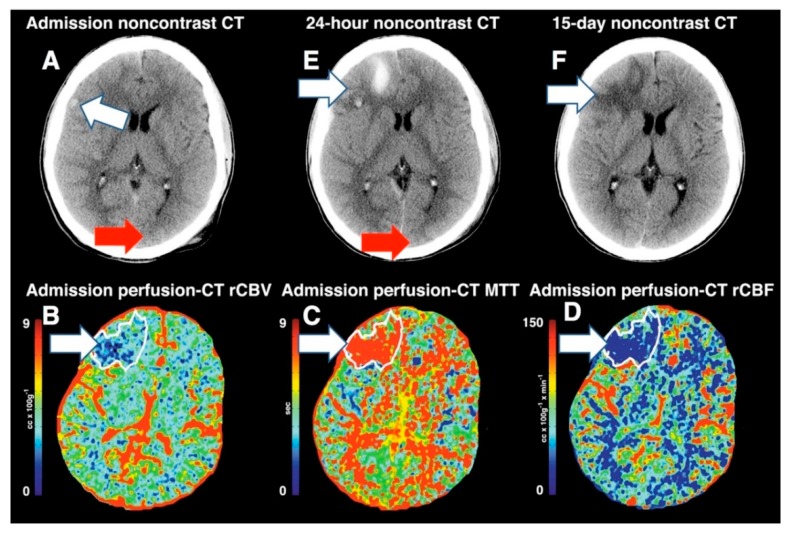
Noncontrast and perfusion computed tomography (PCT) images from a patient with severe traumatic brain injury (TBI). (A) Noncontrast CT at admission revealed a small hemorrhagic contusion in the right frontal lobe (arrow). Admission PCT images demonstrates a large territory of decreased regional cerebral blood volume (rCBV) (B), increased mean transit time (MTT) (C) and decreased regional cerebral blood flow (rCBF) (D). Follow-up noncontrast CT at 24 h (E) demonstrates increased areas of hemorrhagic contusion in the right frontal lobe where the perfusion abnormality was seen. Follow-up noncontrast CT at 15-days (F) demonstrates evolving hemorrhagic contusion and encephalomalacia in the right frontal lobe, which corresponds to the same distribution that is seen on the perfusion-CT on admission. Reprinted with permission from [35].
